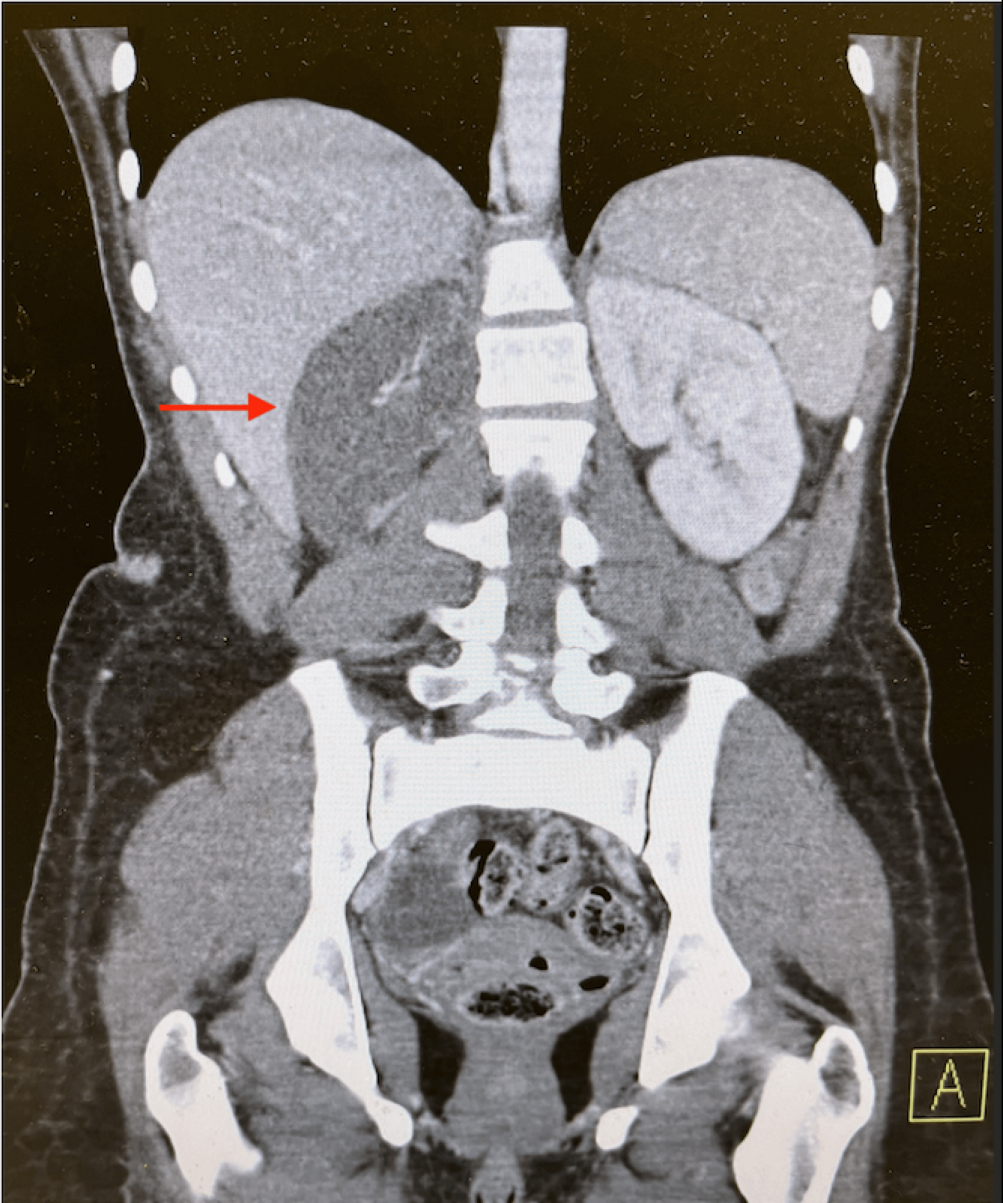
CT scan of the abdomen and pelvis with contrast showing systemic thromboembolic disease with near-global hypoperfusion of the right kidney.
